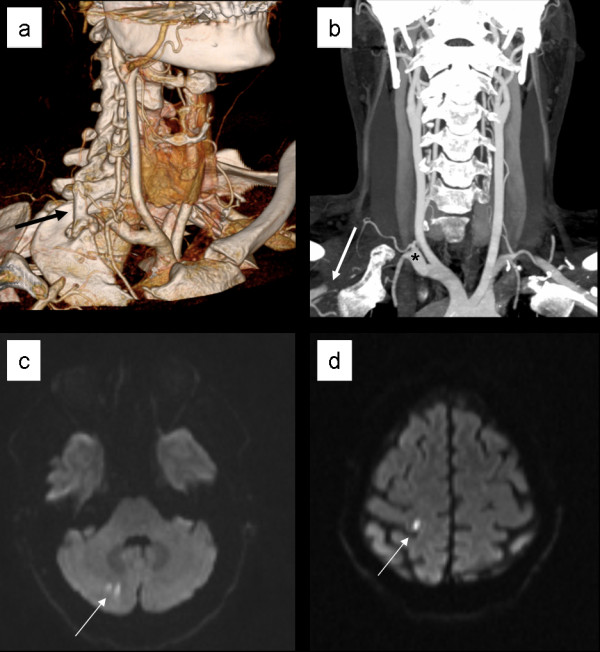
Cervical rib (A) causing subclavian arterial thrombosis (A, B) and cerebellar (C) and cerebral infarctions (D). Computed tomography angiography with surface rendering demonstrates a cervical rib (A black arrow) causing subclavian arterial thrombosis on the right side. The right clavicle has been subtracted from the image. Reformation with maximum intensity projection of the same volume. The right subclavian artery (B asterisk) is occluded proximal to the thoracic outlet and refilled with contrast by collateral vessels before entering the axilla (white arrow). Diffusion imaging (B1000) reveals punctuate foci of acute infarctions in the right cerebellum in C and posterior right frontal lobe in D (white arrows).
